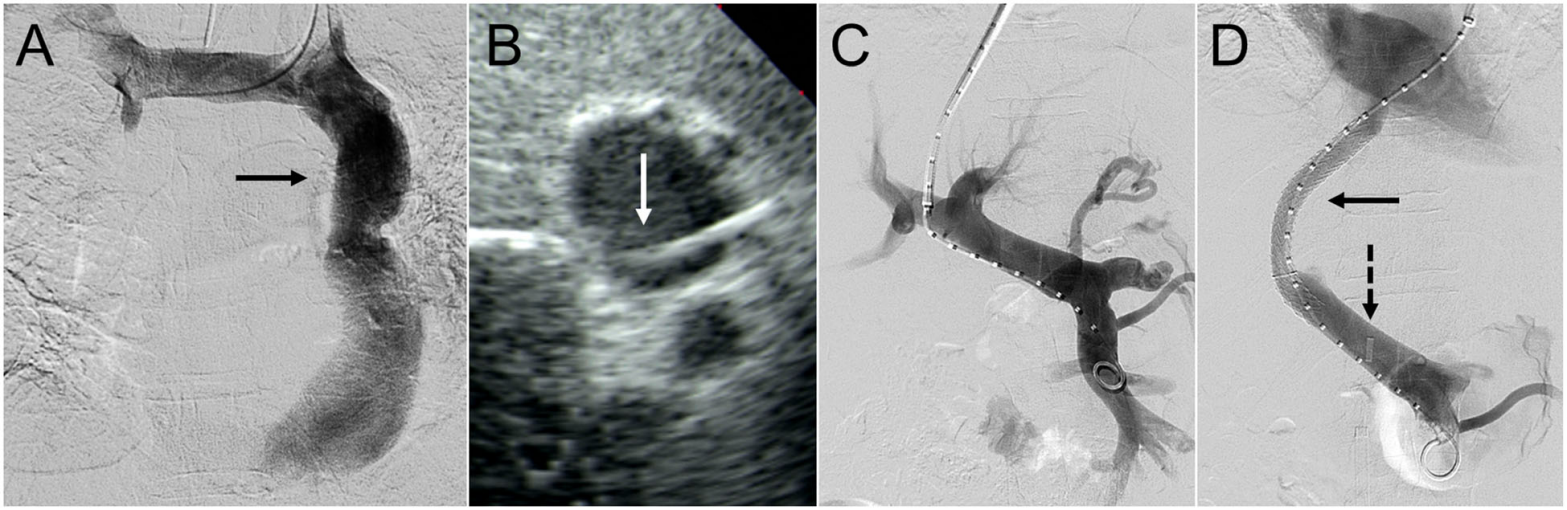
A middle-aged man with end-stage liver disease, hepatitis C, recurrent ascites, and isolated persistent left superior vena cava. a Left internal jugular venography demonstrating the isolated persistent left superior vena cava (solid arrow). b Perpendicular-projecting intravascular ultrasound-guided access to the right portal vein (solid arrow). c Portal venography was performed. d A 10-mm × 6-cm (covered) / 2-cm (uncovered) Viatorr stent-graft was deployed (solid arrow) resulting in widely patent TIPS. A perpendicular-projecting intravascular ultrasound probe (dashed arrow) is shown
